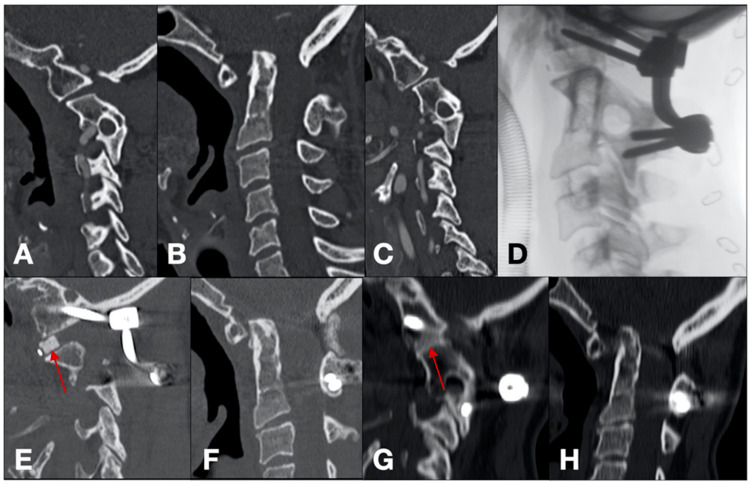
Pre-operative sagittal CT scan (A–C). Intra-operative radiographic acquisition (D). Three-month follow-up CT scan demonstrating correct intra-articular cage positioning (red arrow) and reduction in the malformation (E,F). 58-month follow-up CT scans demonstrating C0/C1-C2 arthrodesis achievement (red arrow) and mild loss of reduction (G,H).
